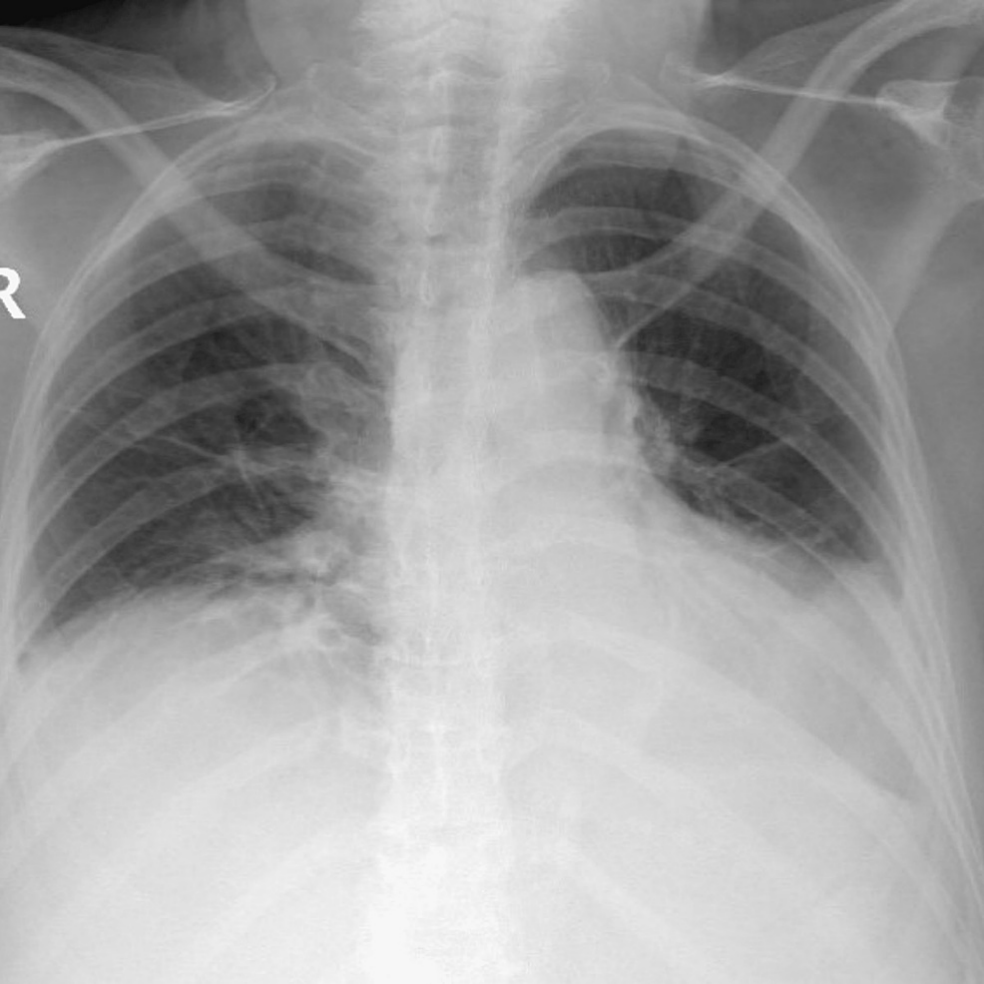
Chest X-ray at discharge Substantial improvement of pleural effusion after corticosteroid onset
